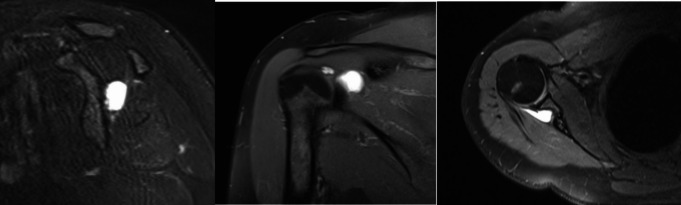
MRI images of paralabral cyst
